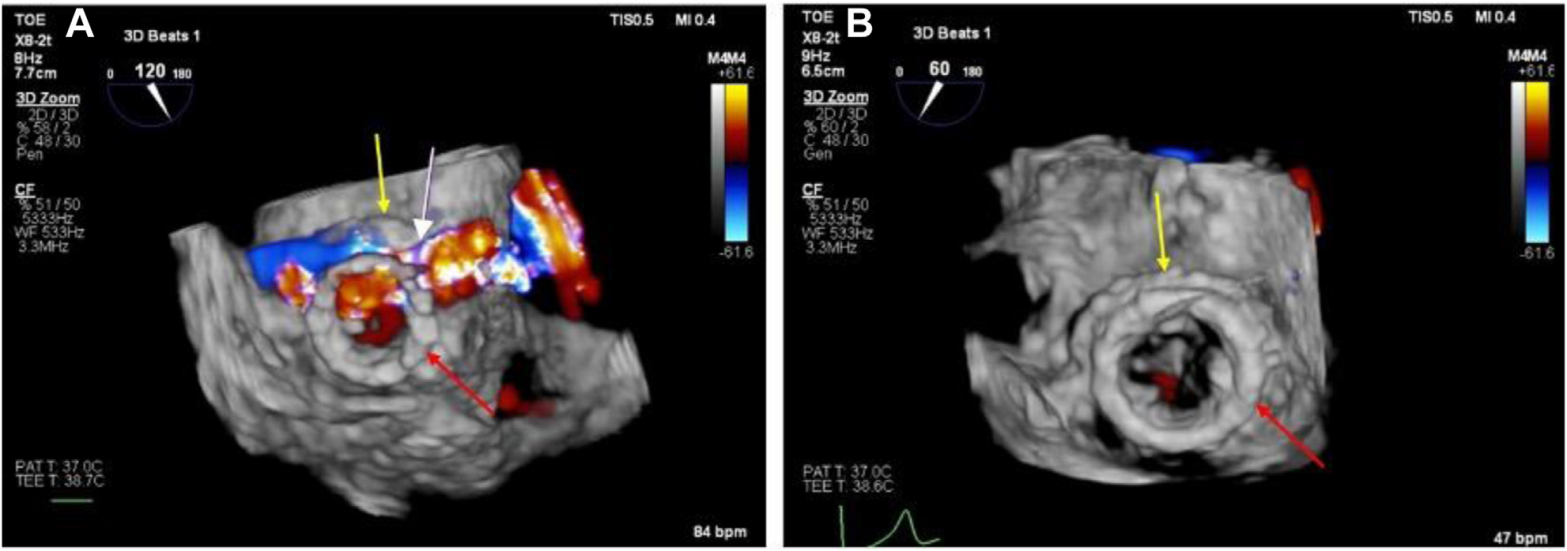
(A) Three-dimensional TEE, volume-rendered reconstruction with color flow Doppler display, en face view of the MV from the perspective of the LA, systolic phase, demonstrates paravalvular and supraskirtal regurgitation (*white arrow*) from the 9 o’clock to 1 o’clock positions arising between 27-mm bioprosthetic MV (*yellow arrows*) and 23-mm ViV (*red arrows*) and (B) resolution of the PVR following implantation of the new 26-mm ViViV.
